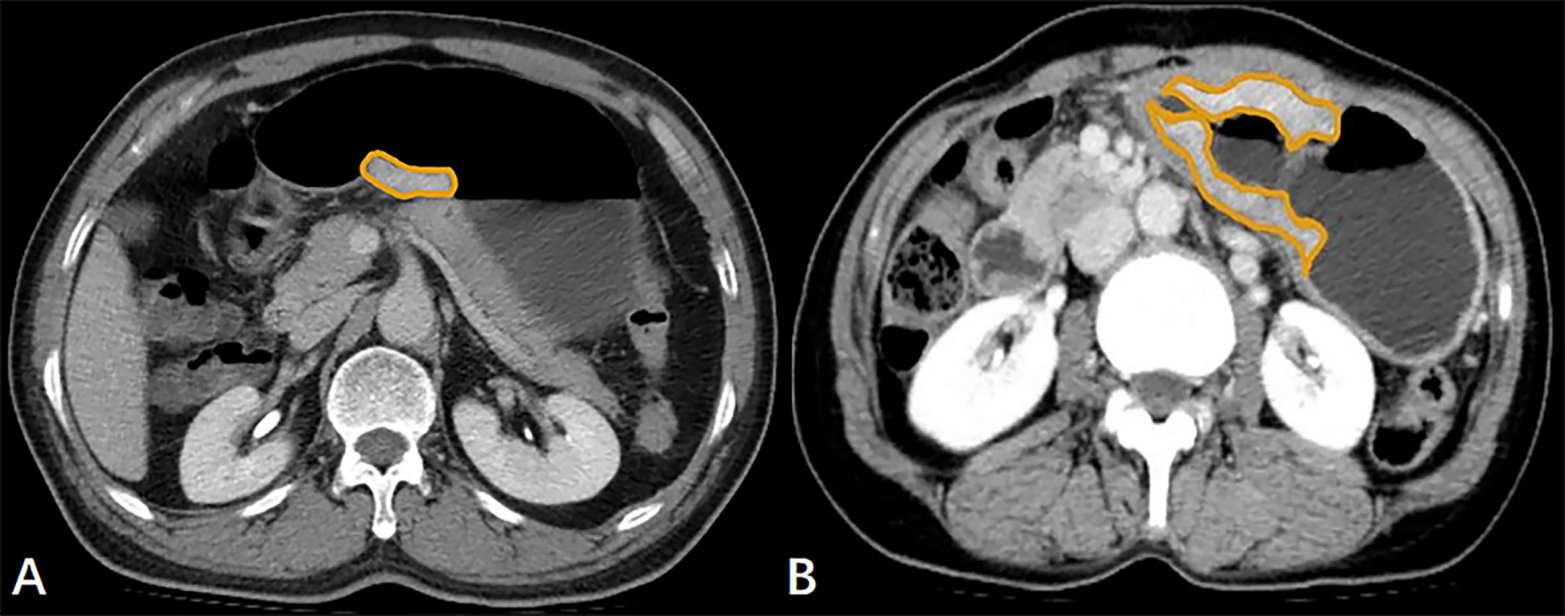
(A) In a 61-year-old male with resectable gastric adenocarcinoma, the initial preoperative abdominal contrast-enhanced computed tomography scans depict the gross tumor volume (GTV) obtained by manual sketching along the margin of the lesser curvature of the thickened stomach wall slice-by-slice, and the GTV is 9.94 cm^3^. (B) The transverse contrast-enhanced computed tomography scans in a 62-year-old female with unresectable gastric adenocarcinoma with lung metastasis show that the tumor area is manually drawn along the margin of the thickened gastric wall, and the GTV is 39.10 cm^3^.
